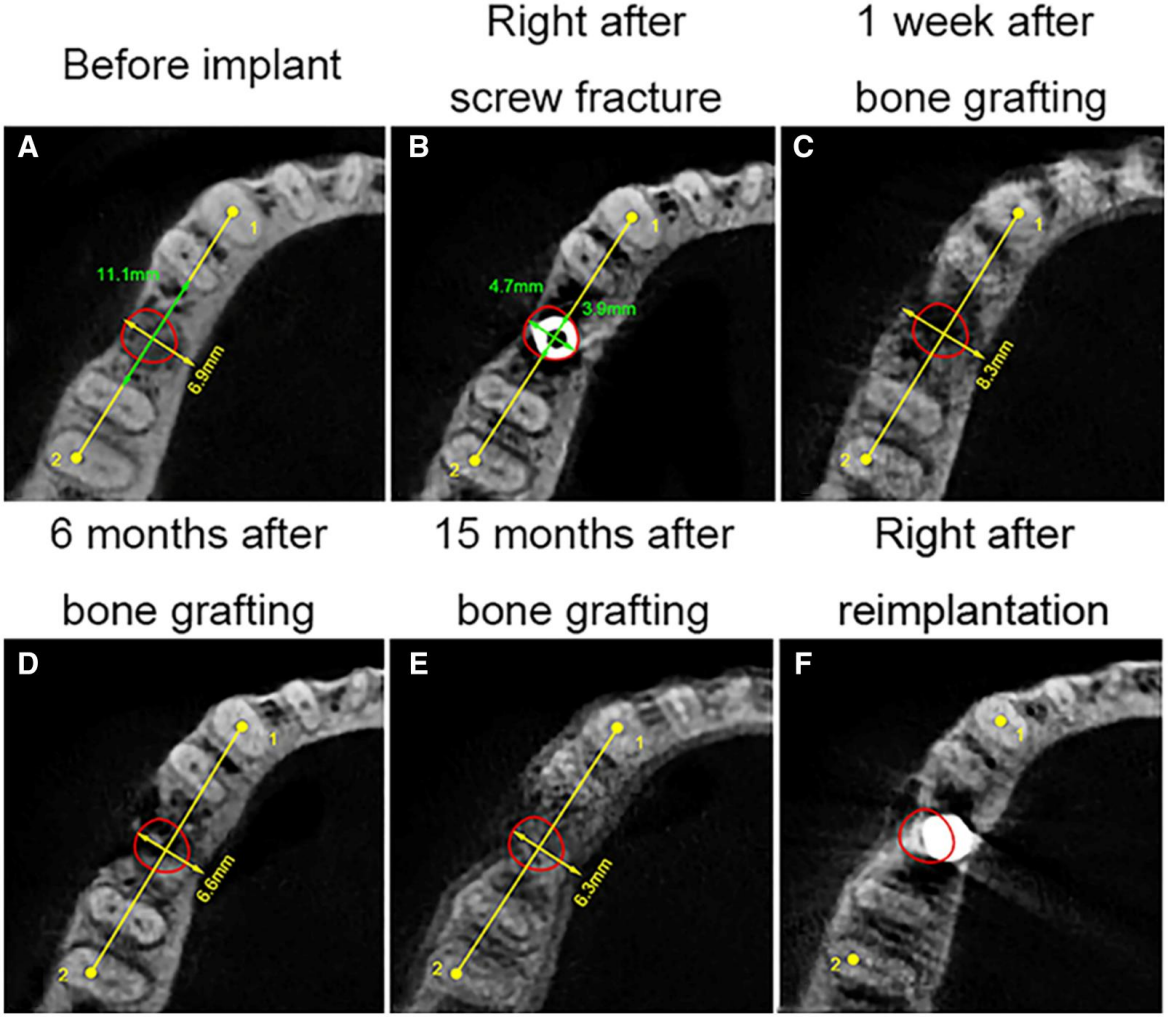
Horizontal CBCT sections at different time points: (A) before implant, (B) immediately after screw fracture, (C) 1 week after bone grafting, (D) 6 months after bone grafting, (E) 15 months after bone grafting and (F) immediately after reimplantation. The red oval shows the bone defect.
